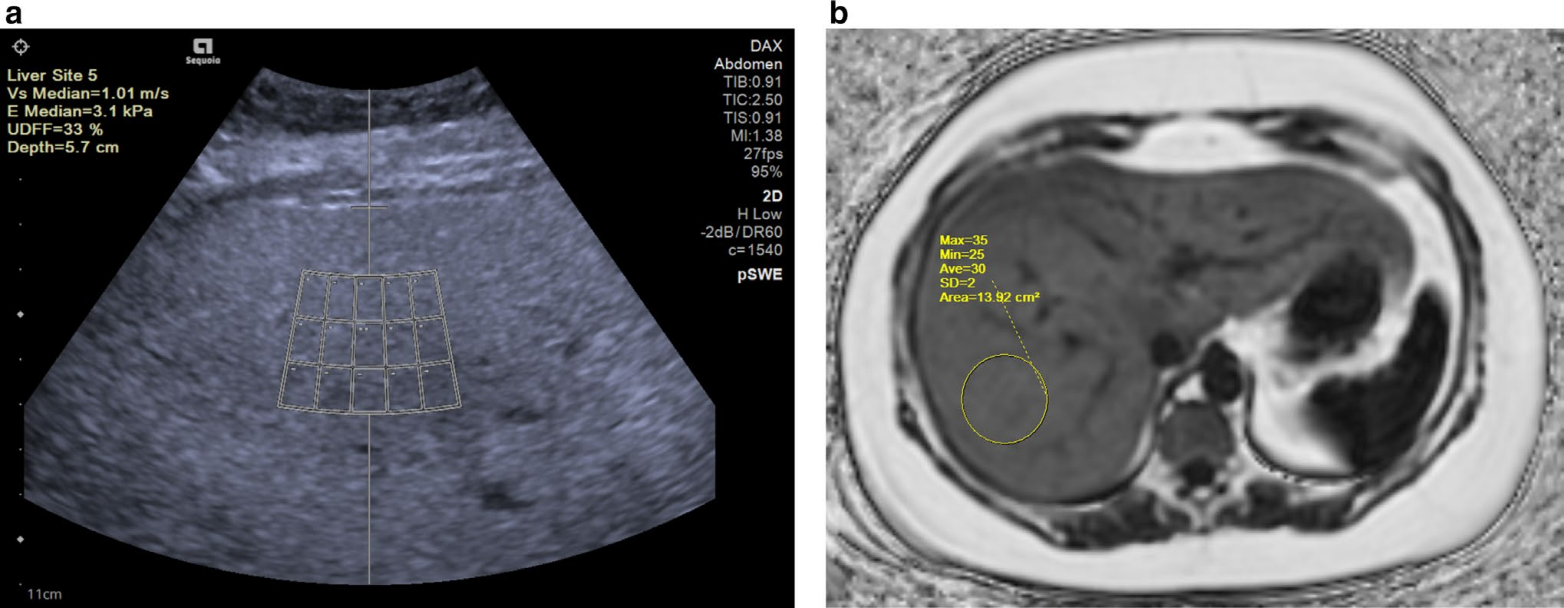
(a) Ultrasound‐derived fat fraction (UDFF) image of a 16‐year‐old girl shows that the liver is diffusely echogenic. The UDFF measured 33%. (b) Axial PDFF MR image of the same patient measures 30% in the posterior right lobe.
